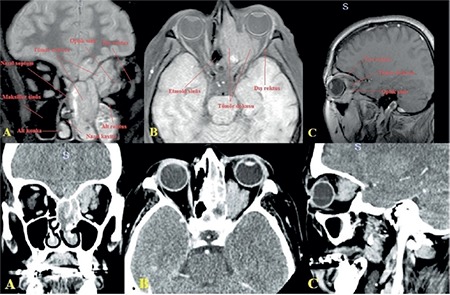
Magnetic resonance and computed tomography images: A mass is seen completely filling the left nasal cavity and anterior/posterior ethmoid sinuses, extending beyond the medial rectus muscle into the cone and displacing the optic nerve inferiorly in coronal (A), transverse (B), and sagittal (C) images
